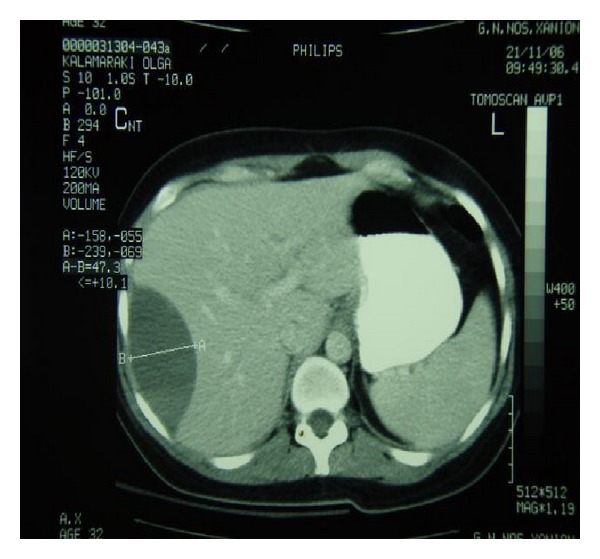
Follow-up CT scan showing decrease of the dimensions of the hematoma.
